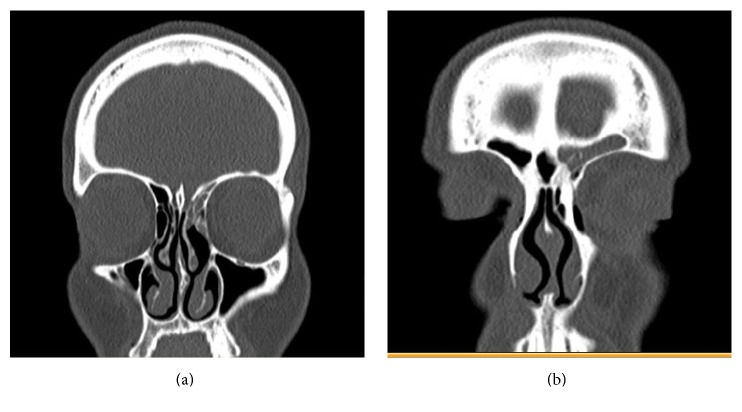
Preoperative coronal CT scan demonstrating evidence of soft tissue density consistent with known inverting papilloma in ethmoid (a) and frontal (b) sinuses.
